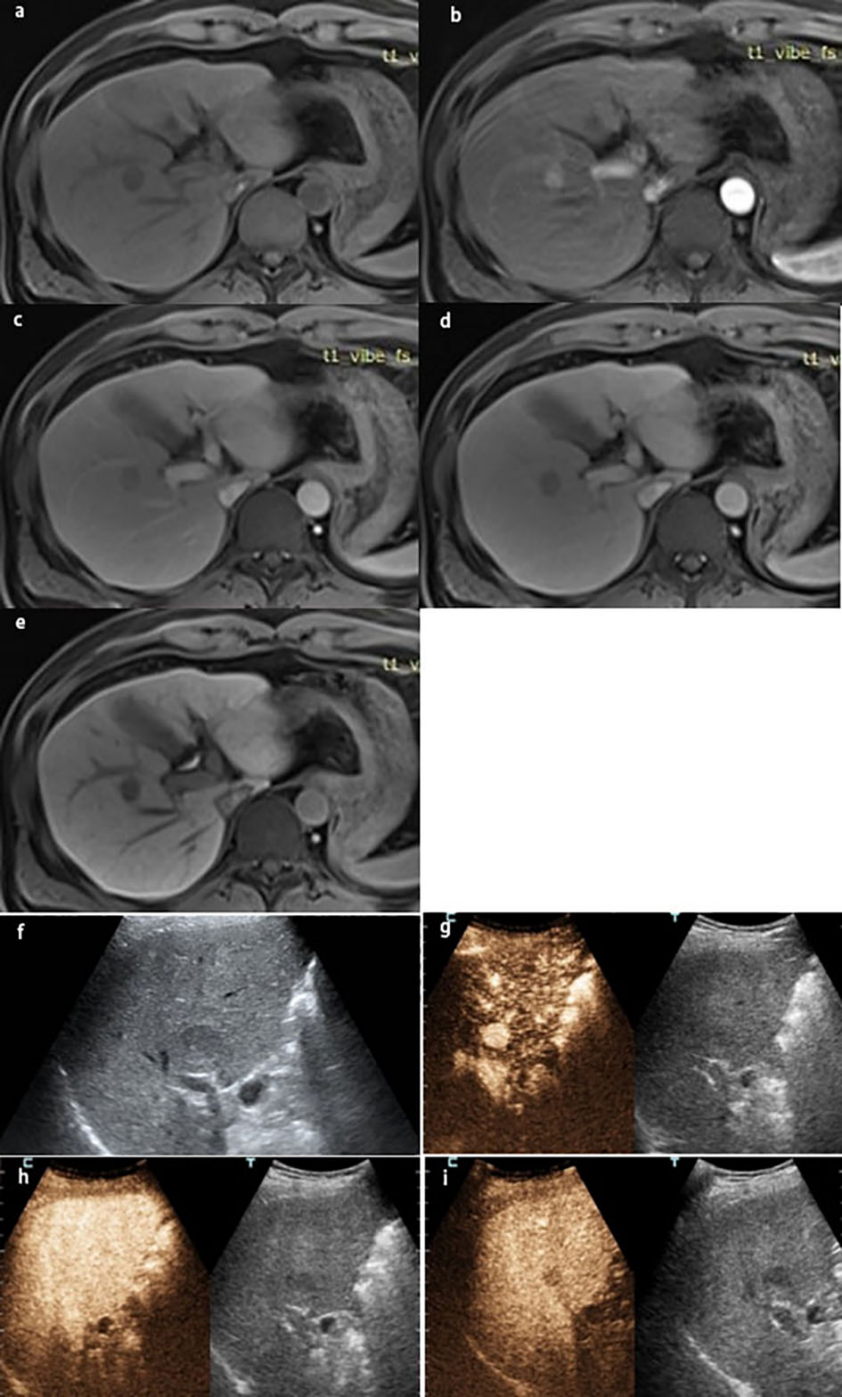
A lesion in S7-8 with a size of 1.7×1.6 cm in a 43-year-old man with chronic HBV infection and cirrhosis. The lesion was classified as LR-5 by EOB-MRI: (A) the lesion was hyperintensity on T1WI, showing (B) arterial phase hyperenhancement, (C) washout on portal venous phase, (D) hypointensity on the transitional phase, (E) hypointensity on the hepatobiliary phase, and (F) gray-scale ultrasound found a lesion on S7-8. The lesion was classified as LR-5 by CEUS. (G) Arterial phase hyperenhancement; (H) no washout on 60s; (I) the lesion was hypovascular on the delay phase. The pathological result by ultrasound-guided puncture was highly differentiated HCC.
